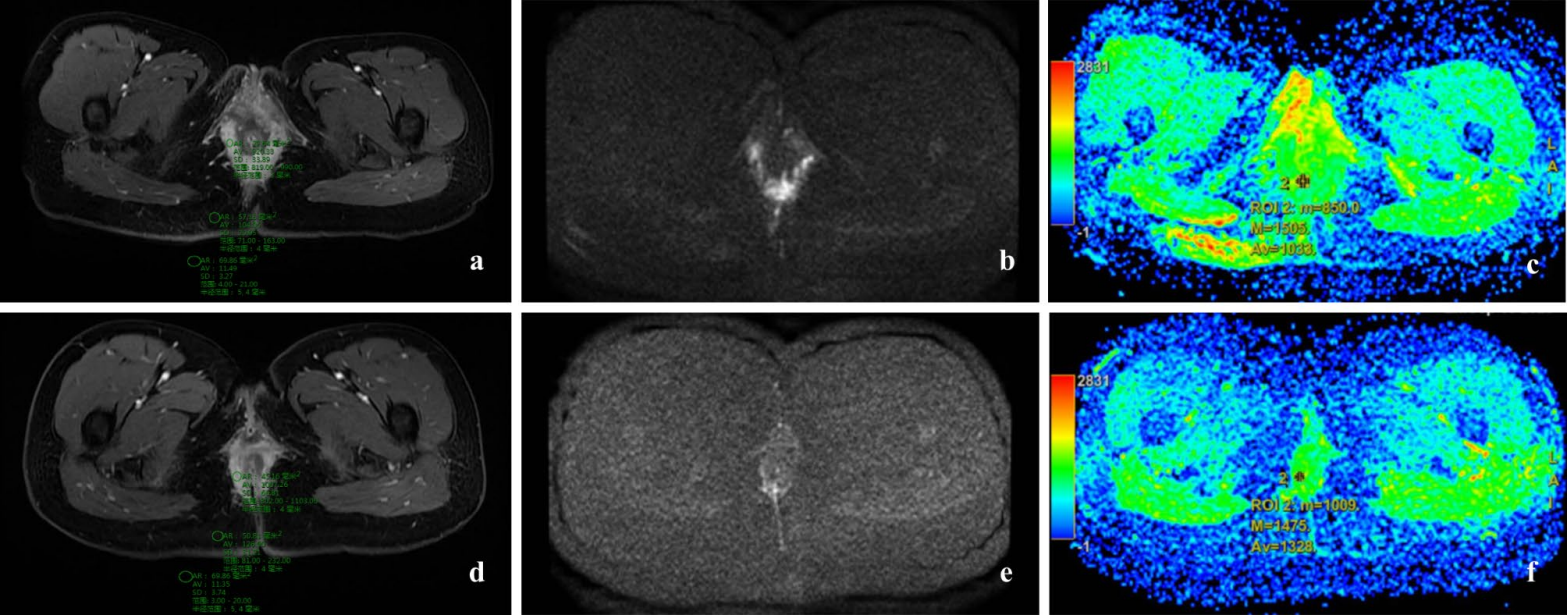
a. The fistula tracks visible on the preoperative DCE images, SI_lesion_, SI_tissue_, and SD_background_ are measured. b. In preoperative DWI in the axial plane (b = 1200), the fistula tracks have a high signal intensity. c. A ROI is manually positioned on the diffusion MR image and copied on the ADC map. d. The same level to measure the SI_lesion_, SI_tissue_, and SD_background_ on postoperative DCE images. e. Postoperative DWI (same level) in the axial plane (b = 1200), showing healed fistula tracks. f. A ROI is manually positioned on the diffusion MR image and copied on the ADC map. DWI, diffusion-weighted images; ROI, region of interest; MR, magnetic resonance; ADC, apparent diffusion coefficient; DCE, dynamic contrast-enhanced; SI, signal intensity
